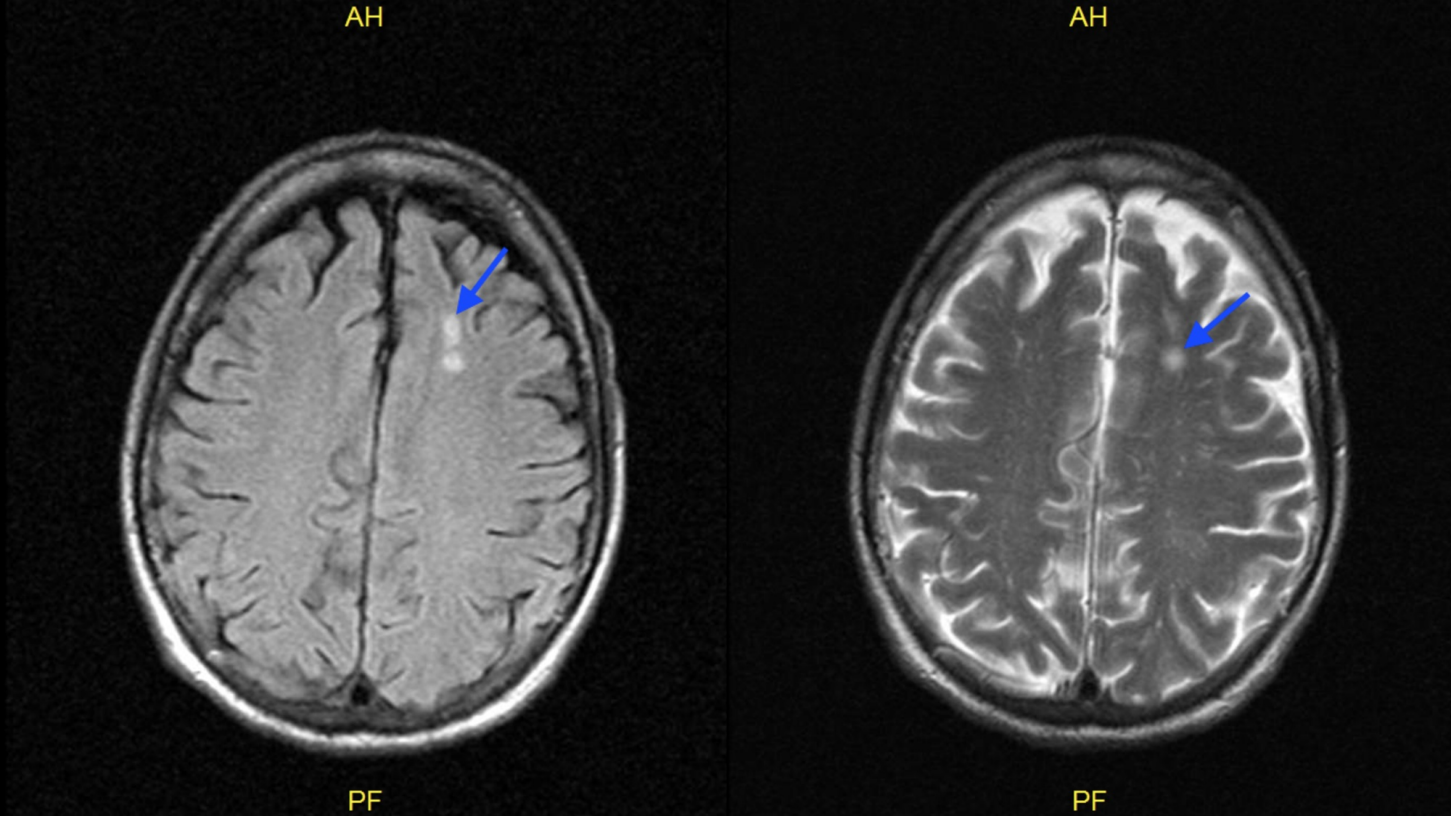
MRI brain without contrast showing nonspecific white matter lesions in the high left frontal lobe measuring approximately 8 mm (blue arrow).
